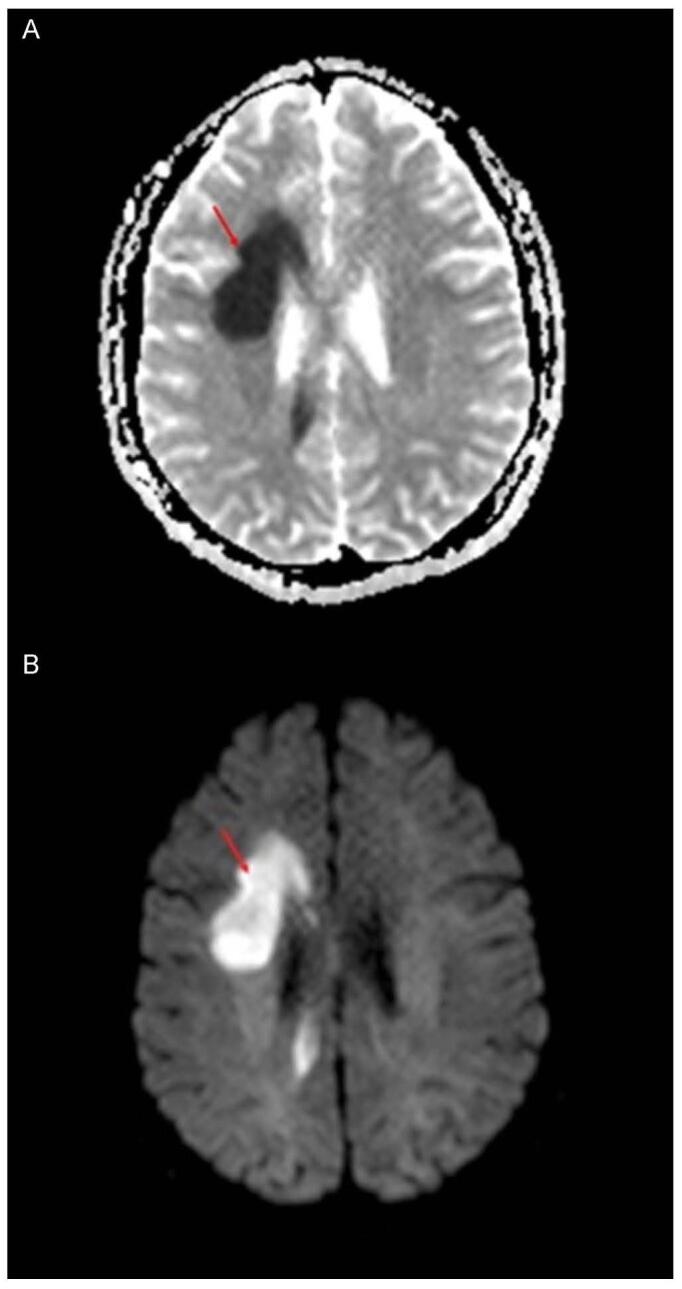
Brain MRI in corona radiata. (A) Diffusion ADC displaying intense restricted diffusion. (B) DWI displaying intense restricted diffusion. Modified from Oarbeascoa et al. (2019). MTX-Induced Subacute Neurotoxicity Surrounding an Ommaya Reservoir in a Patient with Lymphoma^[25]^.
